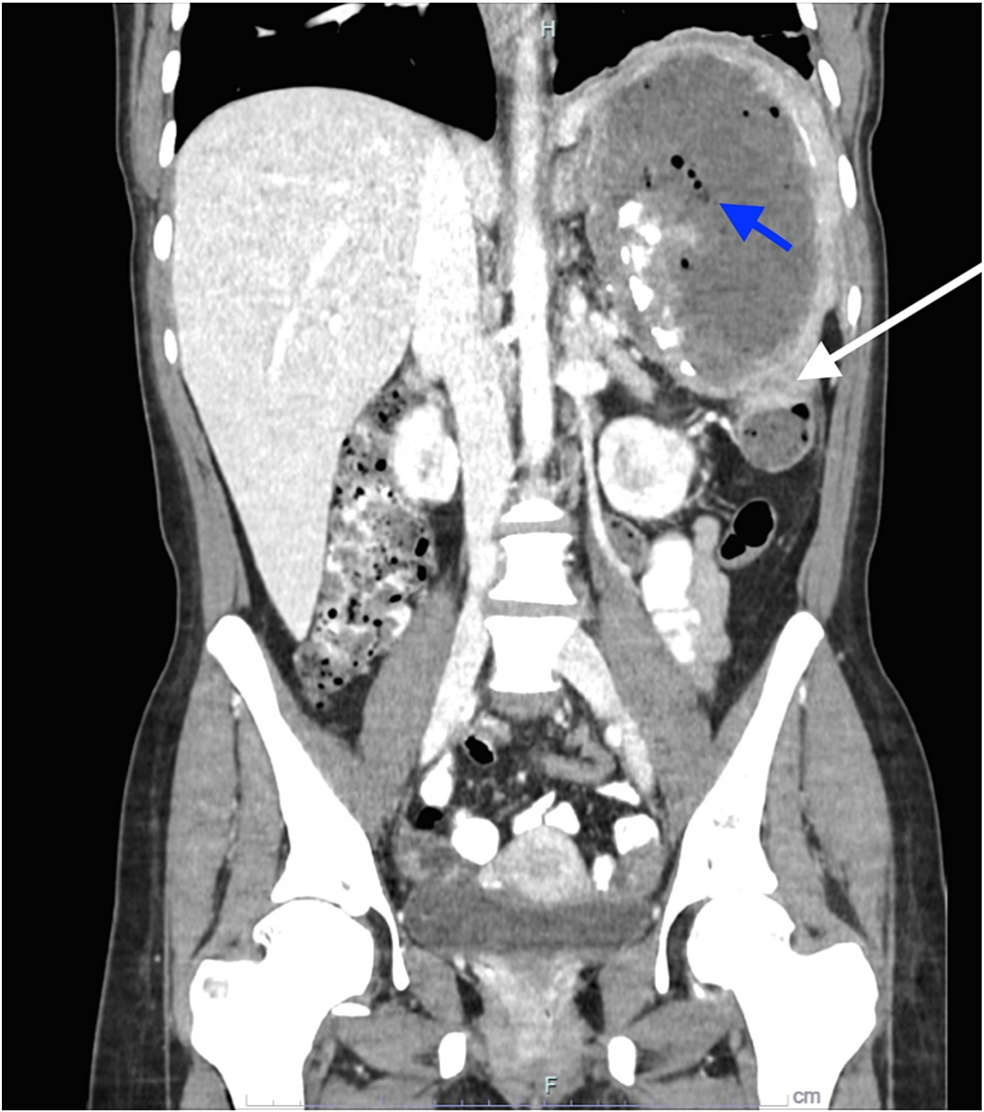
Coronal section of the CT scan of the abdomen showing the entire replacement of the spleen with abscess cavity, air locules in the cavity (blue arrow), and thickening of the peritoneal reflection (white arrow).
